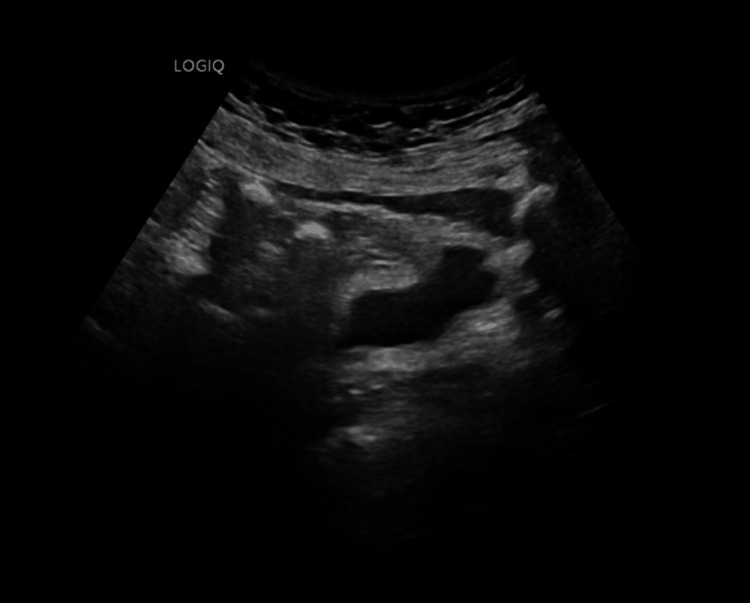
Pelvic ultrasound showing a small, heavily calcified uterus with a visible right ovary.
